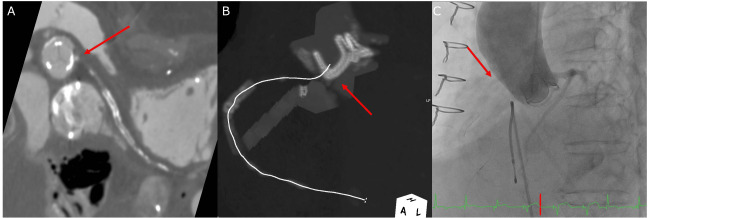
Right coronary artery ostium Coronary computed tomography angiography showing occlusion of the right coronary artery (RCA) ostium (arrow) (A). Maximum intensity projection image showing the RCA ostium close to the stent post of the bioprosthetic valve (arrow) (B). RCA was not contrasted by aortography (C).
